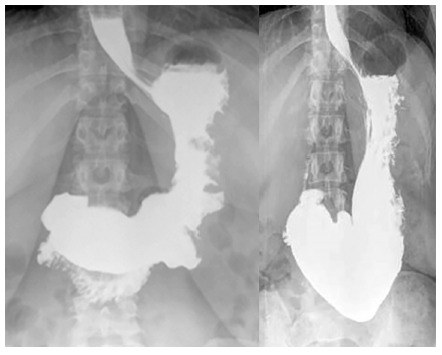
Post-procedure (next day) and 12 month barium radiograph
